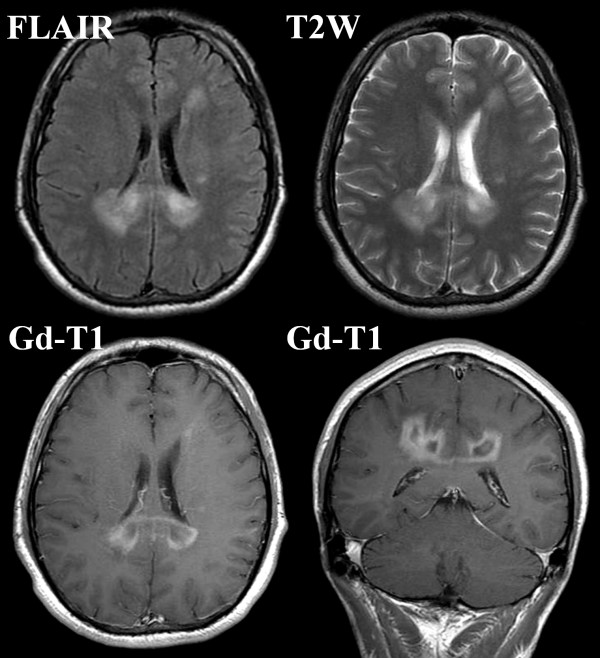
Pre-operative magnetic resonance imaging. High-intensity areas in bilateral occipital forceps majors, the left caudate head, and the left semicentral ovale are shown in both fluid-attenuated inversion recovery (FLAIR) and T2-weighted (T2W) imaging. These lesions are enhanced indistinctly by gadolinium-dimeglumin (Gd).
